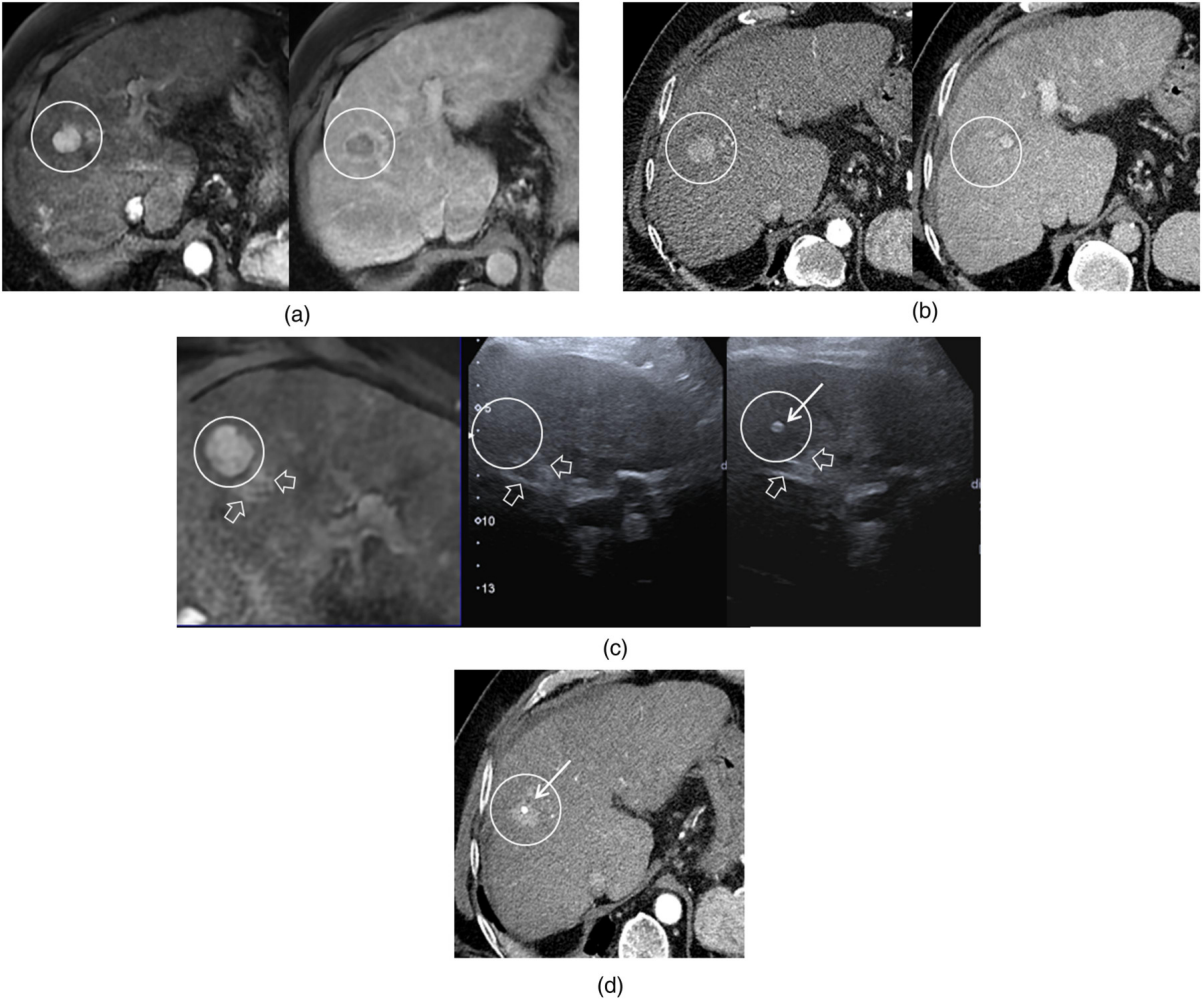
Hepatocellular carcinoma scheduled for thermal ablation. Lesion detection: (a) A 20 mm nodule is clearly seen on T1 MRI during both arterial and portal phases. (b) On CT, the lesion is detected at the arterial phase but not at the portal phase, highlighting potential difficulties for accurate targeting under CT guidance alone. Lesion localization: (c) Fusion imaging with T1 MRI arterial phase failed to directly visualize the nodule on ultrasound. However, adjacent vascular landmarks (hollow arrows) were concordant between MRI and ultrasound, allowing placement of the fiducial marker (white arrow) under ultrasound guidance by targeting the corresponding region. (d) Immediate post-procedural CT confirmed satisfactory marker positioning within the tumor volume.
